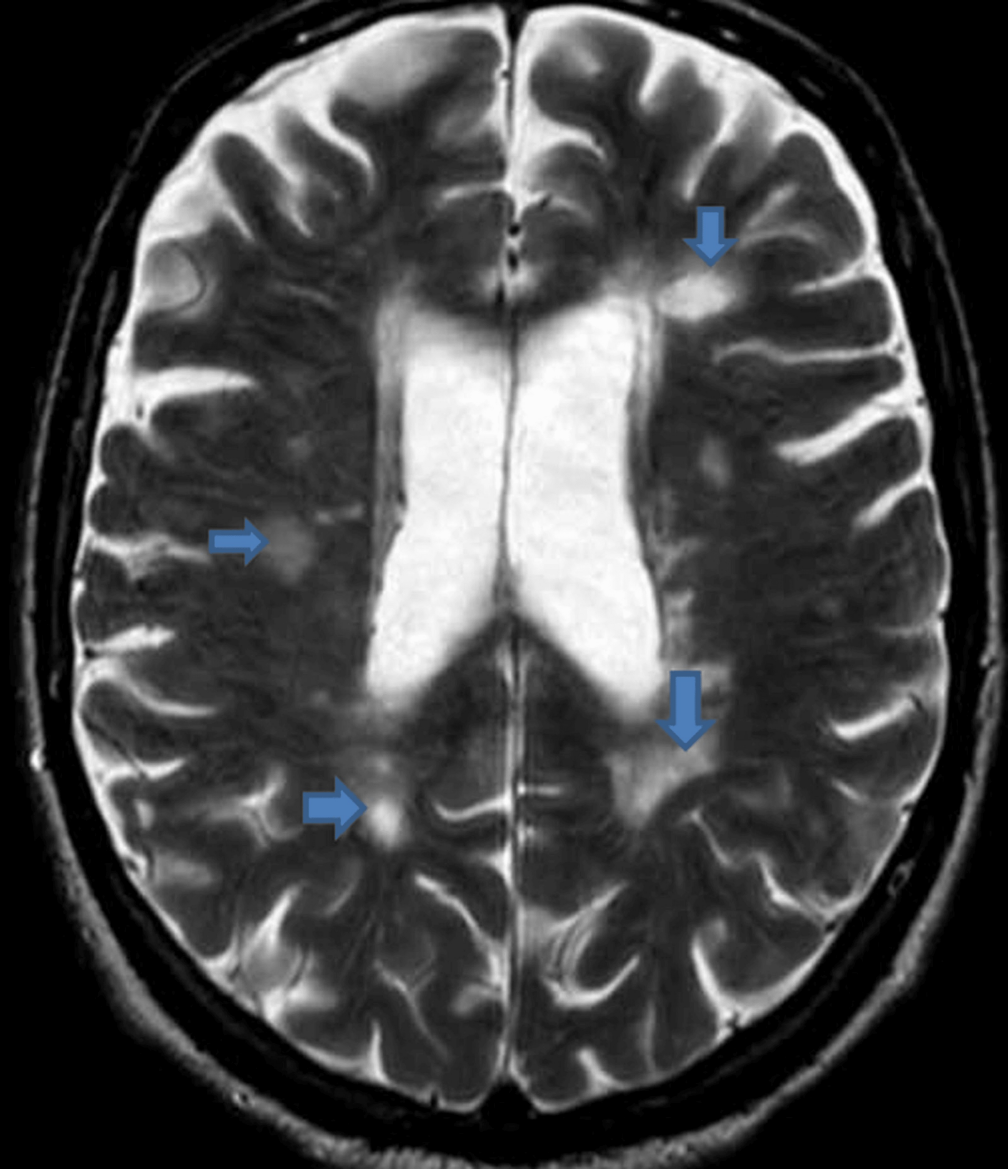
MRI head of the patient, arrows showing multiple demyelinating changes.
